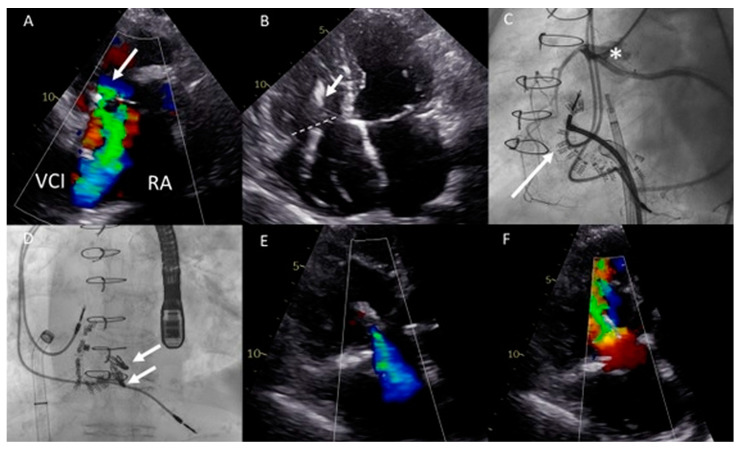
(A) Color Doppler modified parasternal right ventricular (RV) inflow view shows severe tricuspid regurgitation (TR) with a wide vena contracta (arrow) and leakage jet reaching the inferior vena cave (IVC). (B) Apical four-chamber view shows tricuspid valve (TV) annulus dilatation (dash line, 46 mm) and impingement by the RV pacemaker lead (arrow). (C) Left inferior oblique fluoroscopic view of the Cardioband (arrow) annulus reduction procedure (asterisk). (D) Anteroposterior fluoroscopic view shows the two XTW clips (arrows) implanted. (E,F) Modified apical four chamber color Doppler views showing mild (-moderate) residual TR and inflow through the TV (mean gradient of 3 mmHg).
